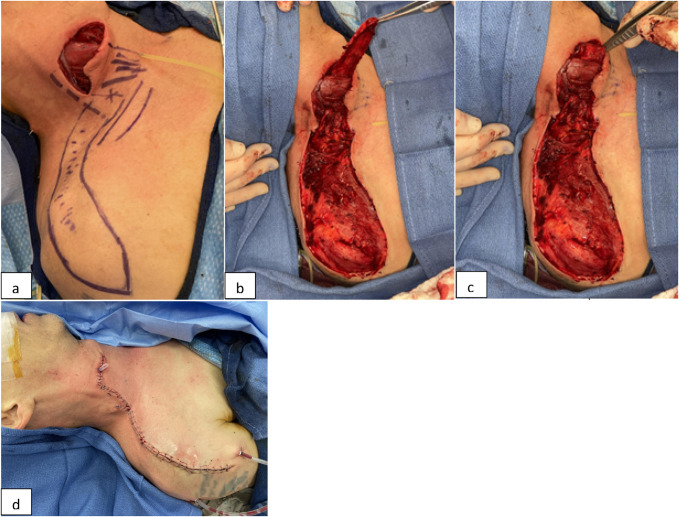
Pictures showing esophageal reconstruction with a SCAIF. A, The triangle between the external jugular vein, clavicle, and sternocleidomastoid muscle was marked, and the pulse from the transverse cervical artery was identified. This pulse was followed laterally into the deltoid region as the supraclavicular branch of the transverse cervical artery. B, An 18-cm long and 5-cm wide flap was harvested and demonstrated good doppler signals. C, The flap was then de-epithelialized and rotated into the paraoesophageal region through a tunnel under the sternocleidomastoid muscle to cover the primary esophageal repair, the great vessels, and the spine. D, The surgical site was closed with drains placed into the paraoesophageal region and the flap donor site. SCAIF = supraclavicular artery island flap
